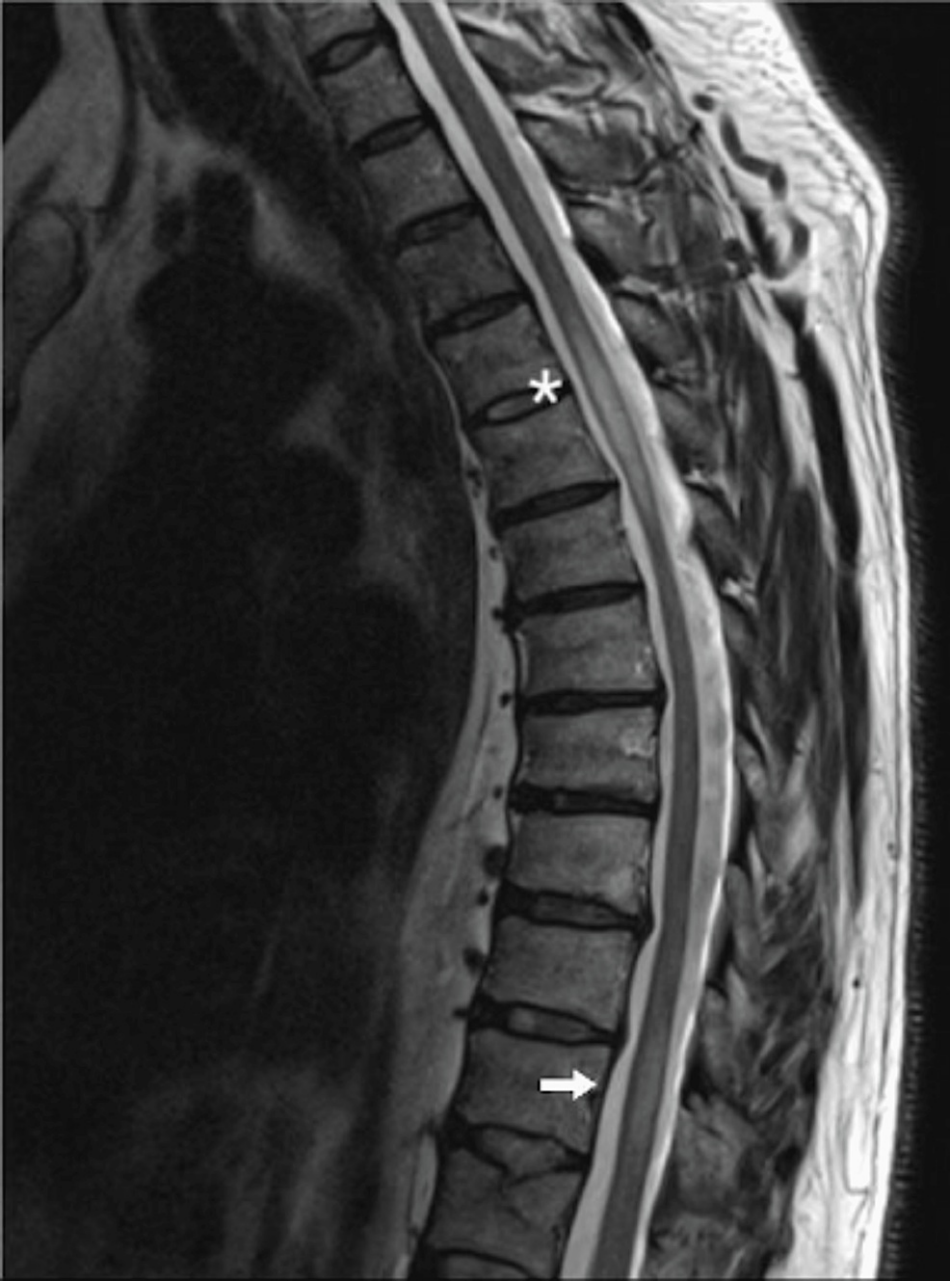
Spinal MRI imaging Spinal MRI T2-weighted image showing hyperintense lesion extending from D4 to D6 (asterisk) and in the transition of D10-D11 (arrow)
